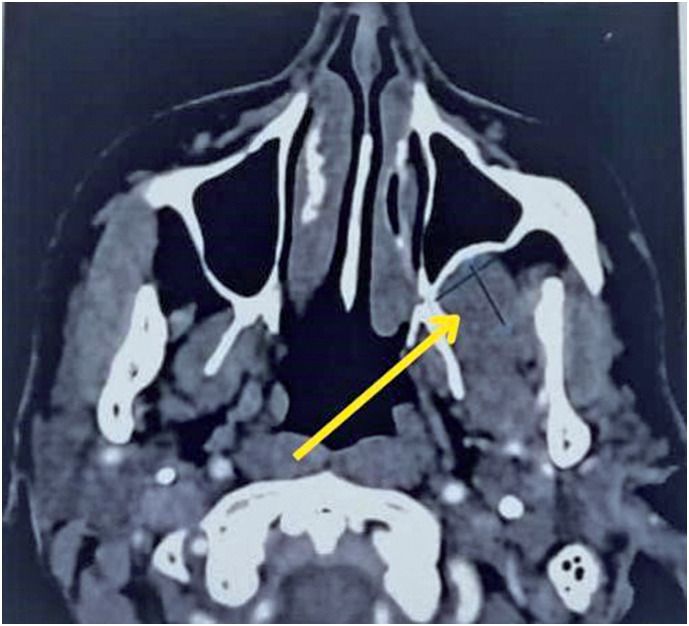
Computed tomography image of the sinuses in a bone window in an axial section, with the yellow arrow indicating a well-defined nodular lesion measuring approximately 2.0cm in diameter
